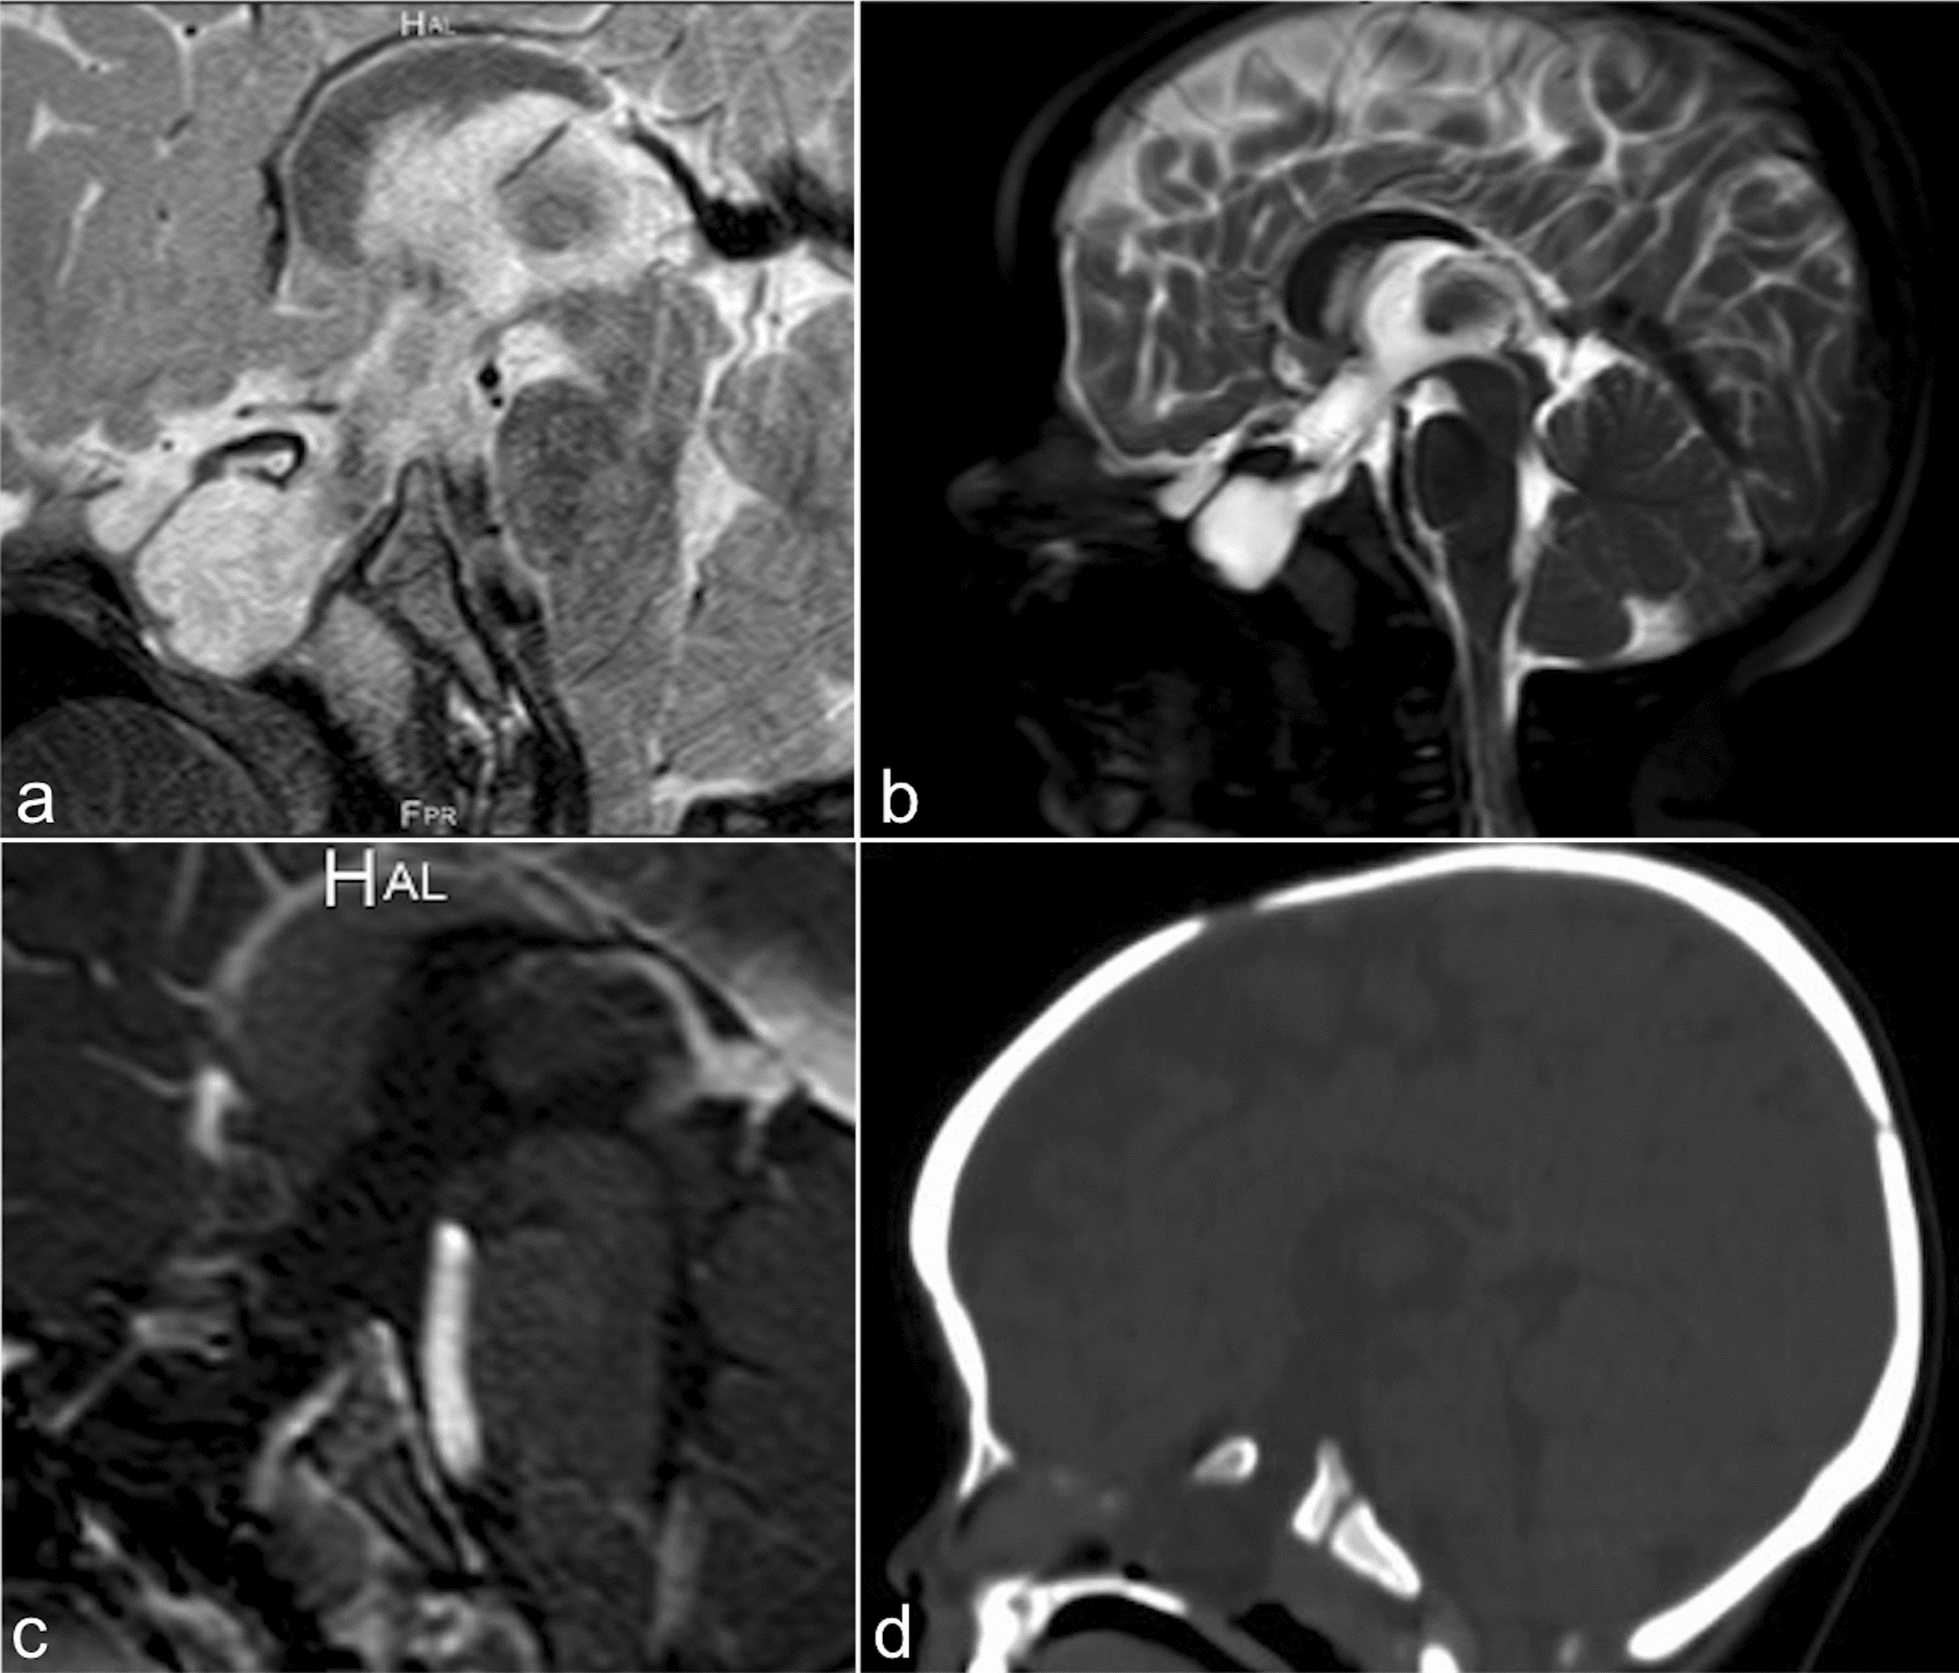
Brain magnetic resonance imaging and computerized tomography. Sagittal T2 (a and b), sagittal post-contrast T1 (c) weighted images, and sagittal non-contrast CT (d) of the brain through midline demonstrate a bony defect at the floor of the sella with herniation of cerebrospinal fluid through the defect into the sphenoid sinuses consistent with a base of skull encephalocele. There is also evidence of absence of the distal body, splenium, and rostrum of the corpus callosum consistent with callosal dysgenesis. The pituitary gland, infundibulum, and optic chiasm were not well visualized raising concern for other associated midline developmental abnormalities
